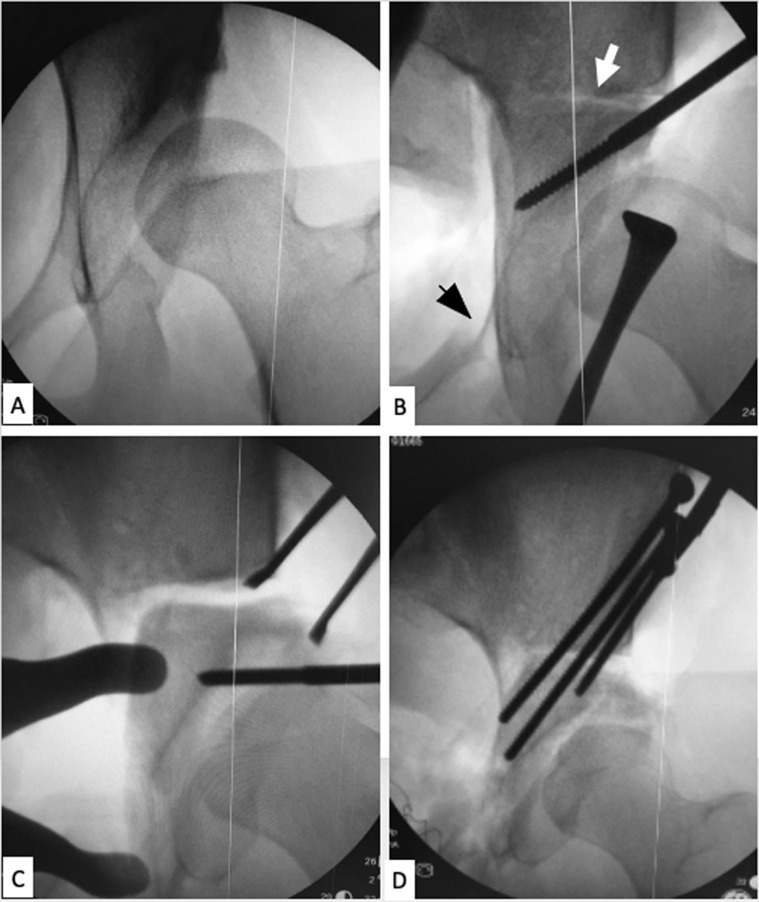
Manipulation of the acetabular fragment, re-orientation and fixation. (A) Preoperative X-ray image. (B) Upon completion of the osteotomy lines. Iliac cut (white arrow) and pubic cut (black arrow) can be seen. A 4 mm Schanz pin can be inserted into the supraacetabular area to help manipulation. (C) Fine tunning the correction with the help of a laminar spreader. (D) Final correction and fixation. Coverage (CEA) has improved with horizontal sourcil.
